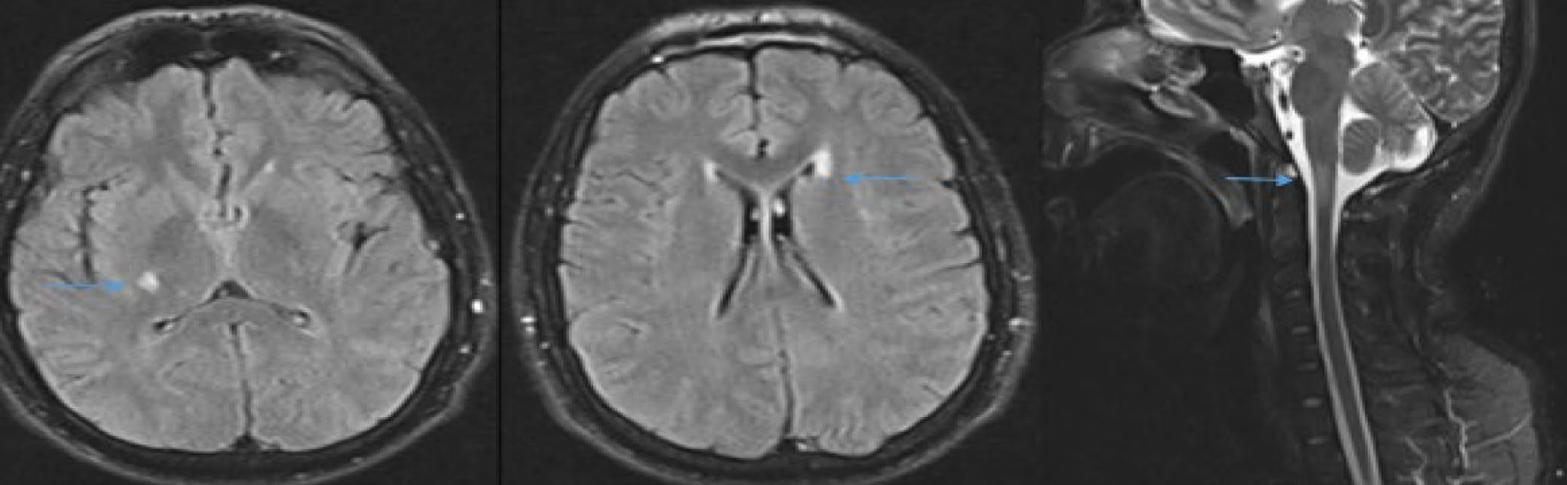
The MRI shows a FLAIR hyperintensity in the right internal capsule (left), sub ependymal periventricular area along the left lateral ventricle (middle), and a STIR cervicomedullary junction lesion in the sagittal image (right).
